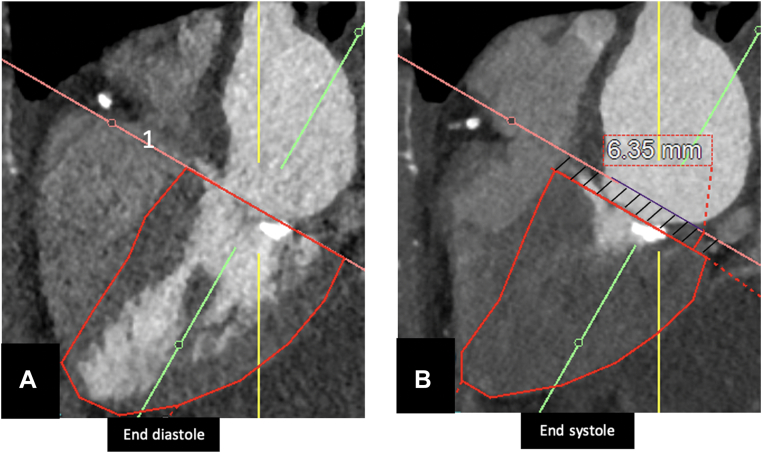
Measurement of Mitral AVPD Using CCT on a 4-Chamber View The AVPD can be seen as a piston-like movement of the AV plane from the base to the apex within the LV. On CCT, AVPD was measured in three long-axis images (4-chamber view, 2-chamber view, and LV outflow track view). The positions of the mitral AV plane in end diastole (A) and in end systole (B) were determined in the 3 views, with the vertical down placement of the MV plane from diastole to systole corresponding to the AVPD height (in mm). The average of the three locations was calculated. AVPD = atrioventricular plane displacement; CCT = cardiac computed tomography.
